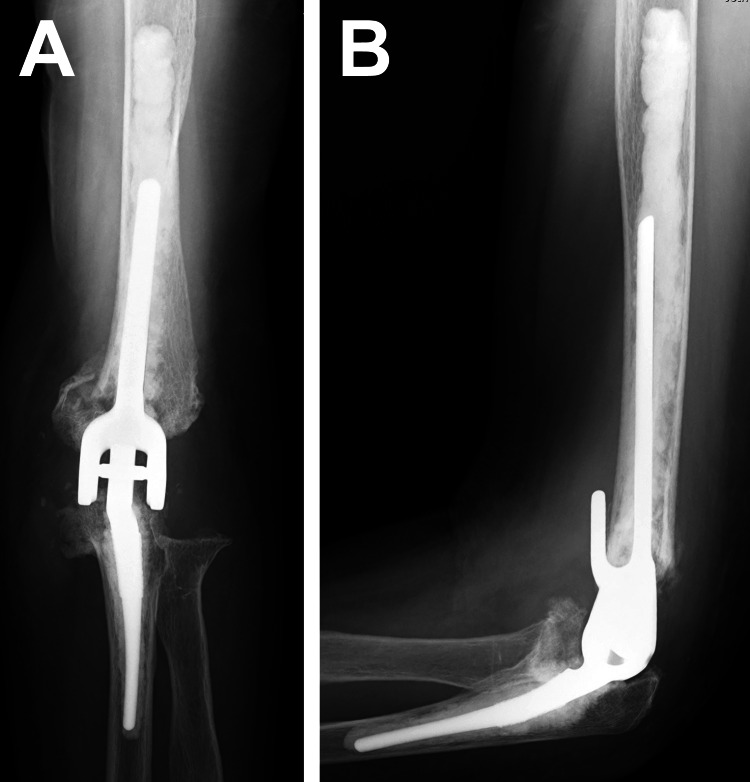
Postoperative radiographs at the one-year follow-up. The anteroposterior image (A) and the lateral image (B).
